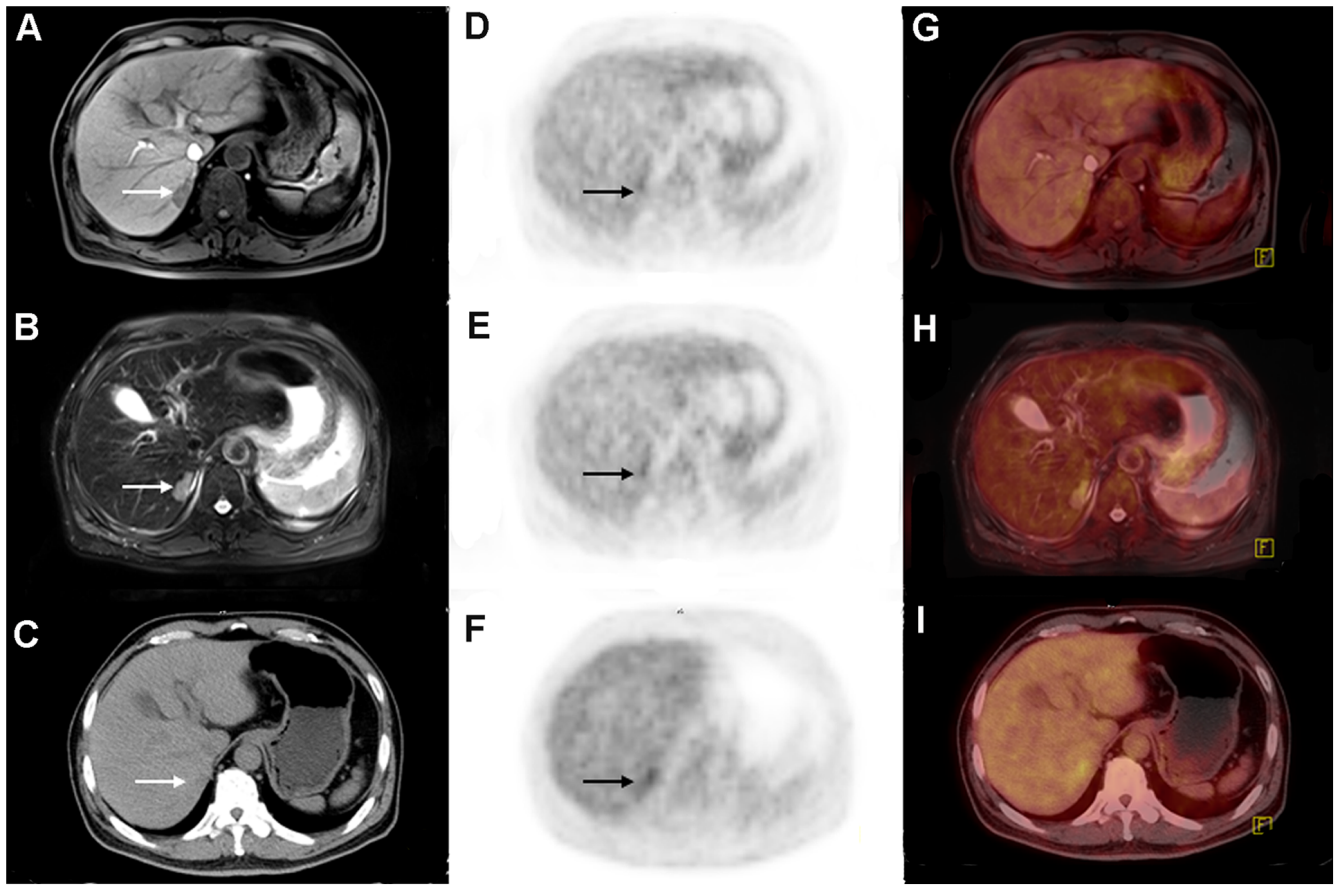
A 62-year-old female with history of breast cancer resection with a solitary liver nodule in follow-up study. T1WI (B), T2WI-FS (D) and CT (F) showed the well-moderate differentiated hepatocellular carcinoma (*arrows*), whereas PET (A, C, and E) did not show abnormal uptake of fluorodeoxyglucose (FDG).
